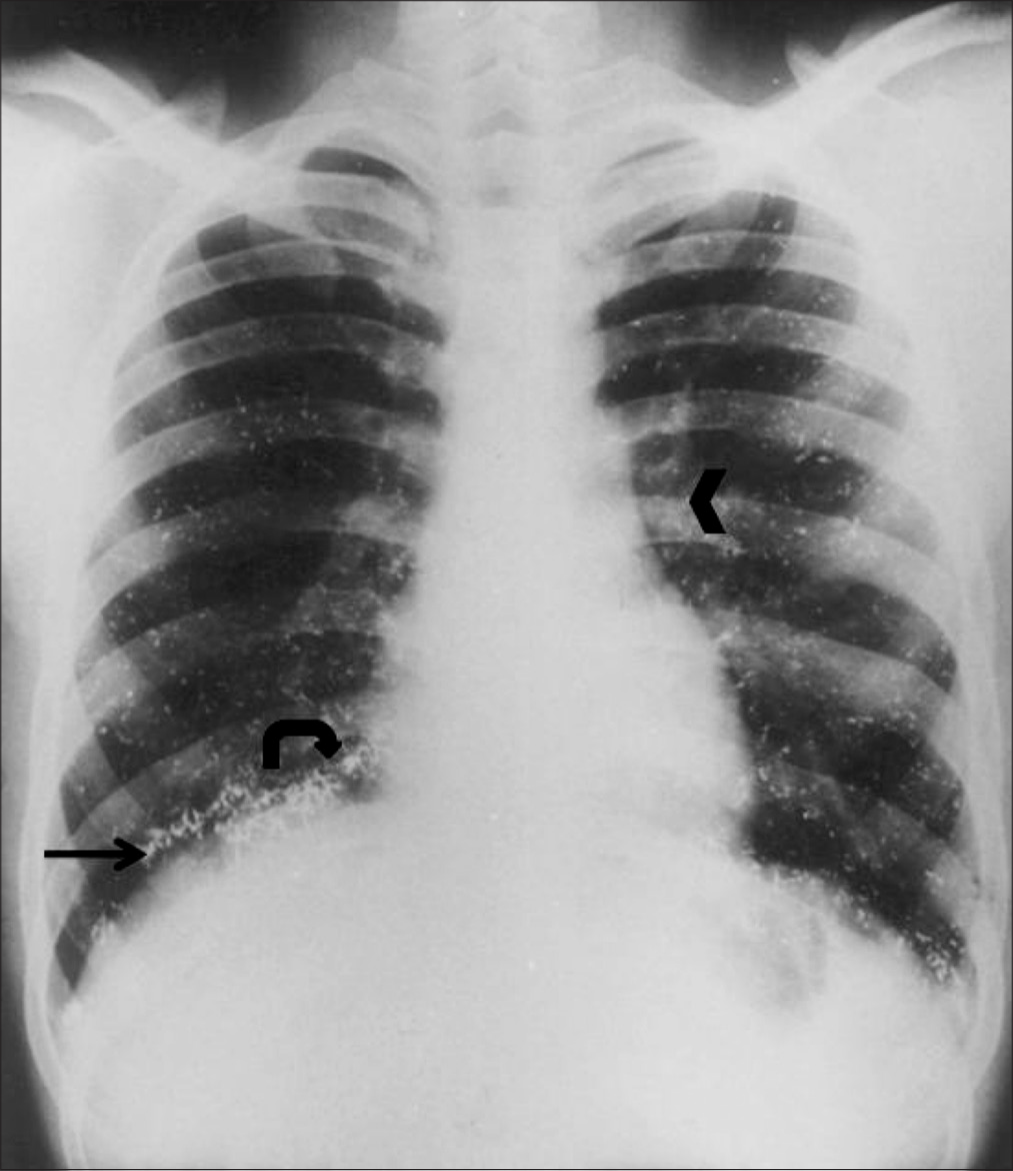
Radiograph of the chest shows multiple tiny metallic densities arranged in a discrete fashion throughout the lung parenchyma bilaterally, predominantly at the lung bases (arrow). Densities are also seen in both hila (arrowhead) and at the cardiophrenic angles (curve arrow)
